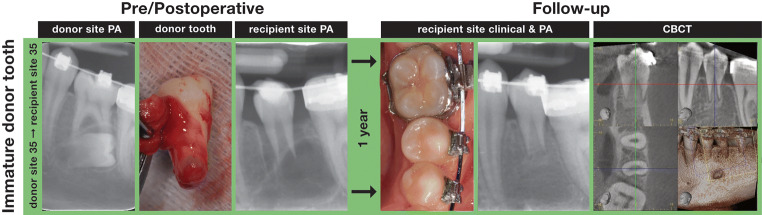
Ingrowth of bone-like tissue into the former pulp chamber is visible in the CBCT of a transplanted lower left second premolar one year after surgery. A “periodontal ligament-like layer” connects this tissue to the dentin walls facing the chamber
